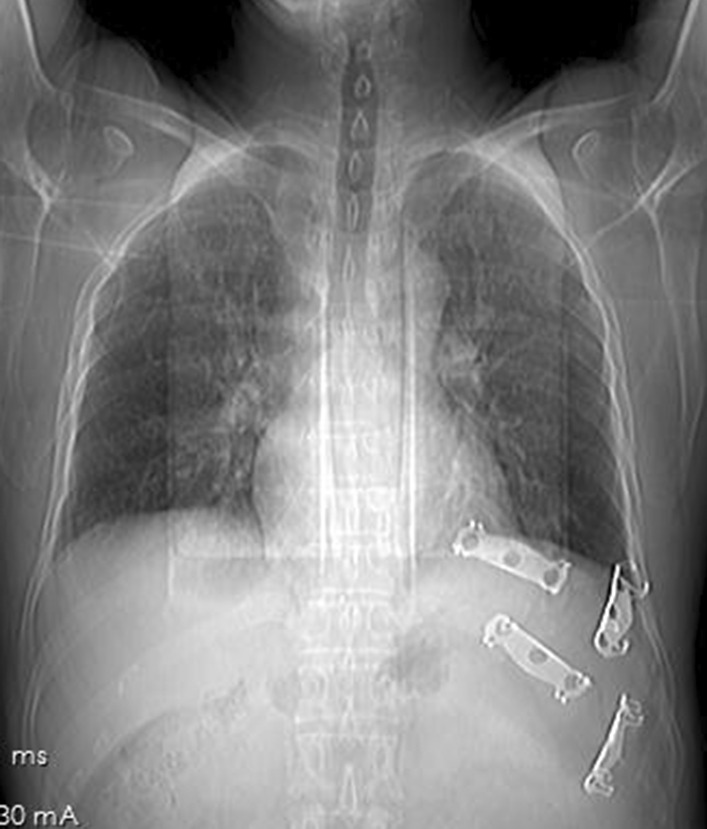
Reexamination of chest CT
